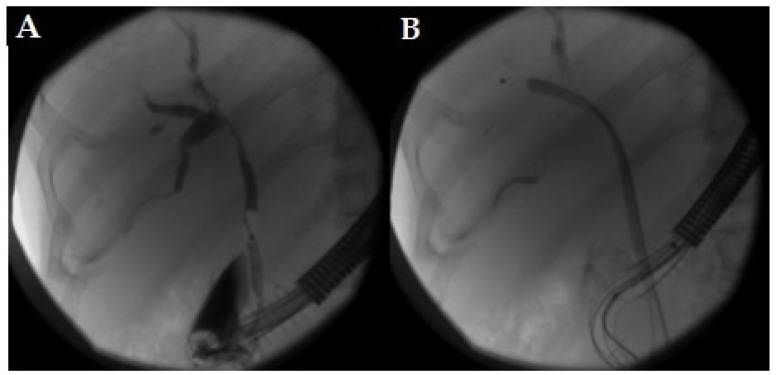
(A,B): X-ray image of an ERCP procedure with Ambu Single-Use Duodenoscope. The patient had a Klatskin tumor. The main bile duct was successfully cannulated (A) and a metal stent was delivered without any technical problems (B).
